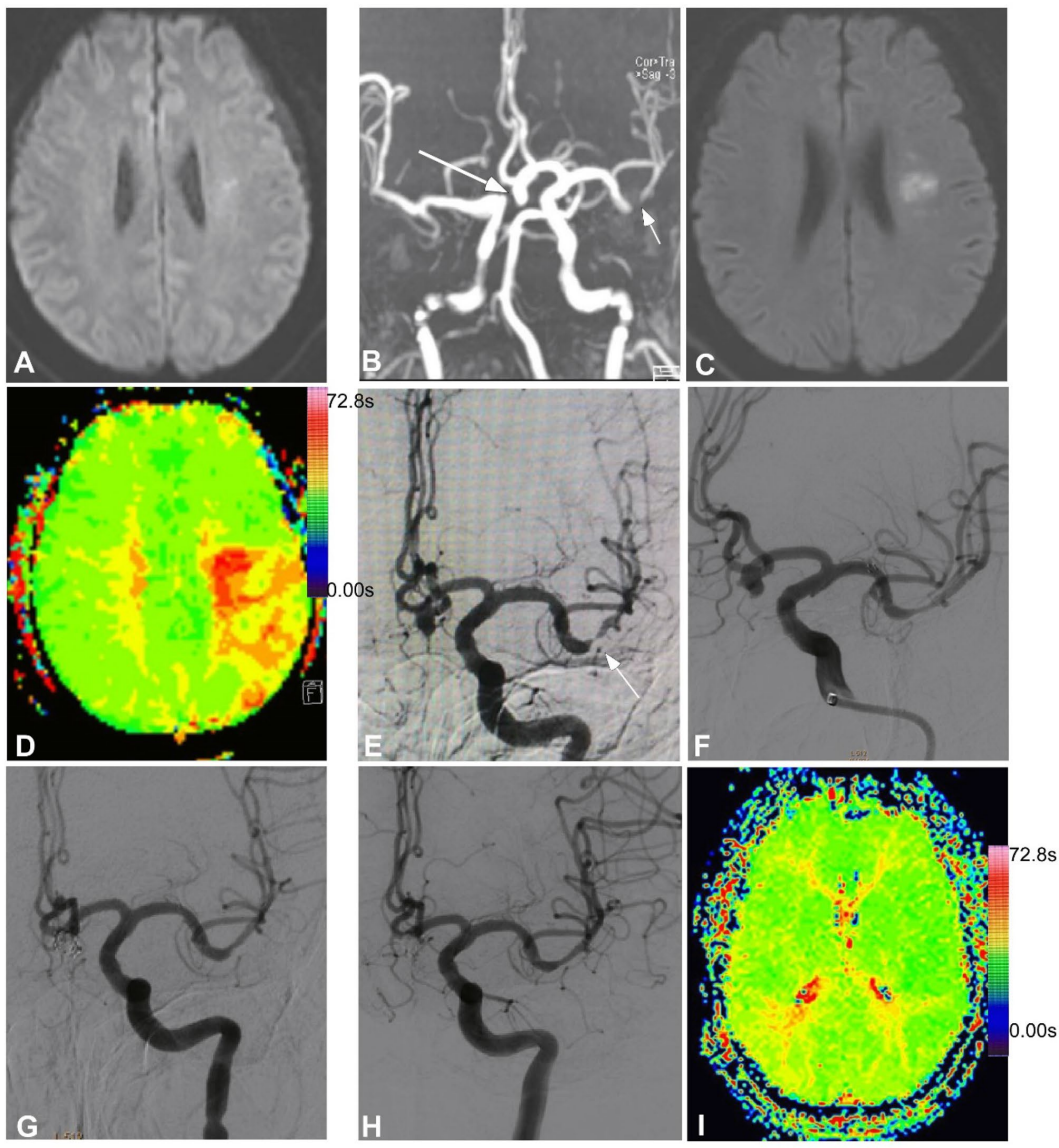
Severe stenosis in the lower trunk of the left middle cerebral artery (MCA). (A) Magnetic resonance imaging (MRI) showed bead-like high intensity lesion near the ventricular body. (B) MRI angiography revealed severe stenosis in the lower trunk of the left MCA (small arrow) and an aneurysm (large arrow) at the anterior communicating artery. (C) Nine days after admission, the symptoms were aggravated and the second MRI scan demonstrated increased infarction area. (D) Perfusion imaging showed that greater time is needed for the blood flow to reach the left parietal occipital lobe. E&F. Digital subtraction angiography demonstrated severe stenosis in the lower trunk of the left MCA (arrow, E), and stent angioplasty was performed with an Enterprise stent to relieve the stenosis (F). G. Endovascular coil embolization was performed to completely occlude the anterior communicating aneurysm. (H). Follow-up angiography at six months following stenting revealed patent flow in the lower trunk of the left MCA. (I). Perfusion imaging showed the time is significantly improved for the blood flow to reach the left parietal occipital lobe.
